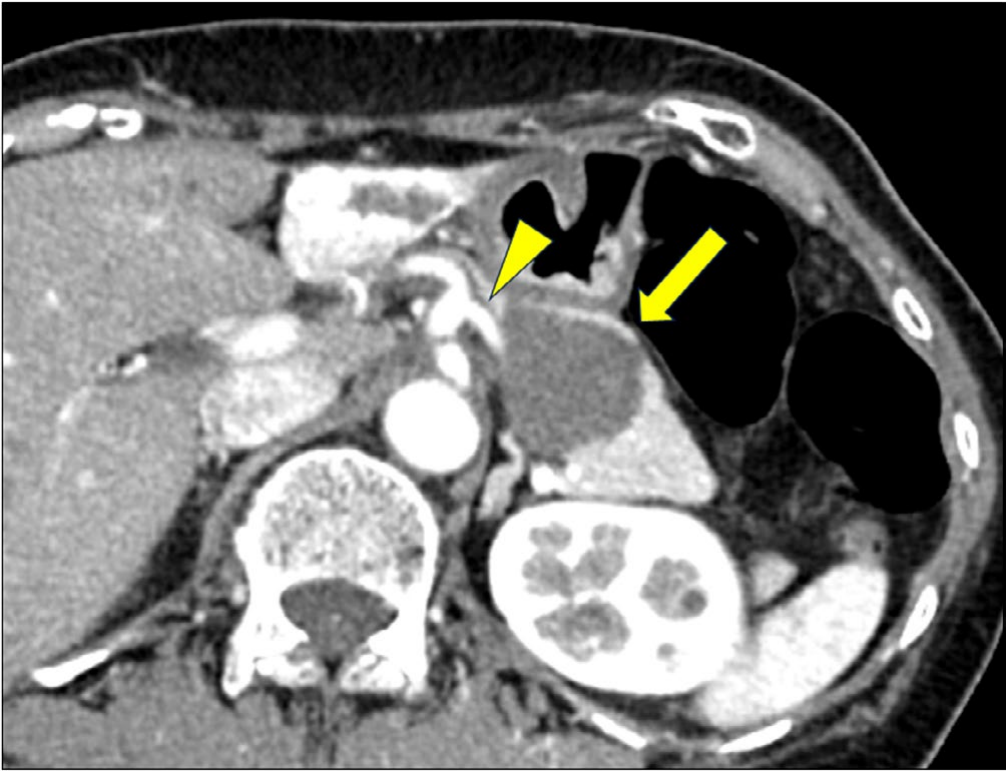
Contrast‐enhanced computed tomography shows a cystic tumor with gradual contrast enhancement in the body of the pancreas (arrow). The tumor was in contact with the splenic artery (triangle).
